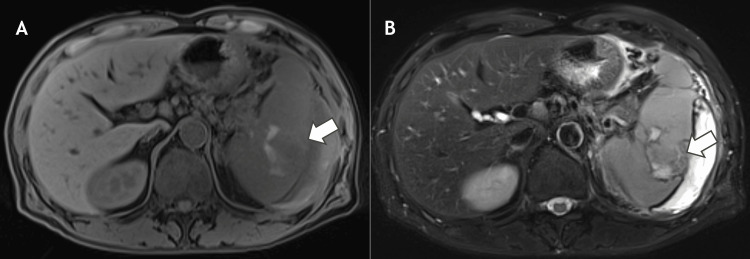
Magnetic resonance imaging findings of the spleen (A) T1-weighted axial image and (B) T2-weighted axial image demonstrating heterogeneous signal intensity within the splenic parenchyma (arrows), raising concern for a possible neoplastic process.
